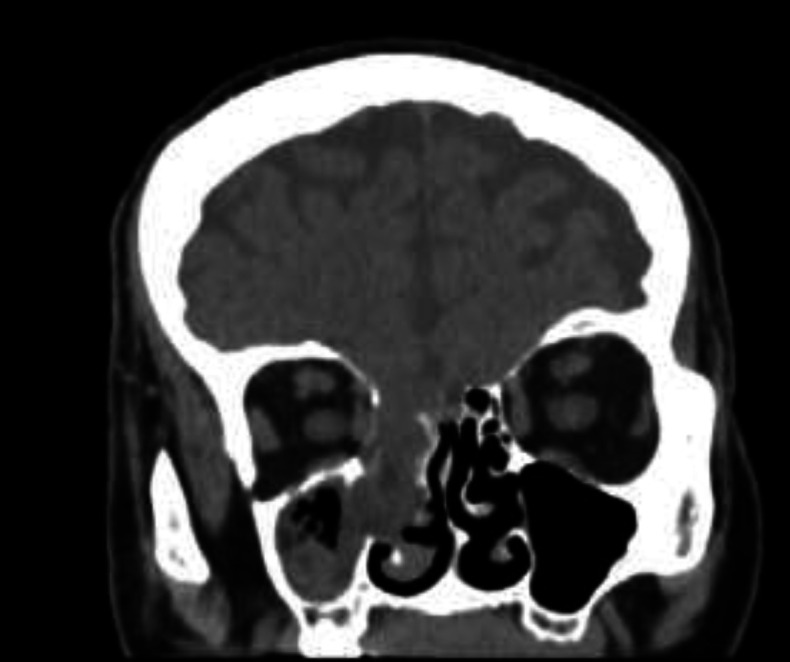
Meningoencephalocele pre-op CT
